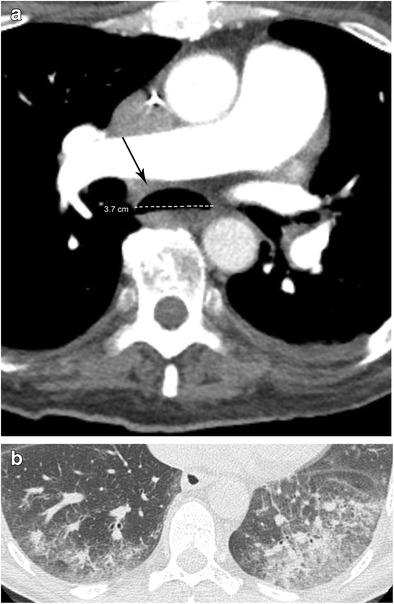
Scleroderma. a CT angiogram of the chest in soft tissue window demonstrates a dilated oesophageal lumen. b Chest CT of the same patient in lung window demonstrates bilateral dependent areas of ground glass opacity and reticulation with relative subpleural sparing observed in the setting of chronic aspiration
